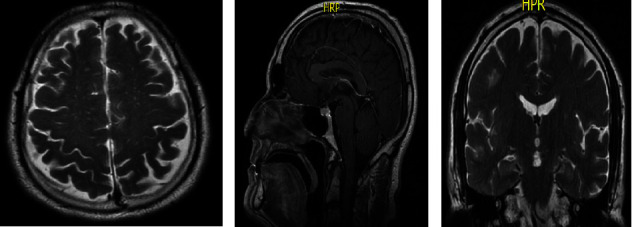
Results of MRI at cortical (a), sagittal (b), and coronal sections at the level of the temporal lobe (c) showing widening of cerebral sulci, subcortical atrophy, and hippocampal atrophy in the patient with advanced Korsakoff's syndrome.
